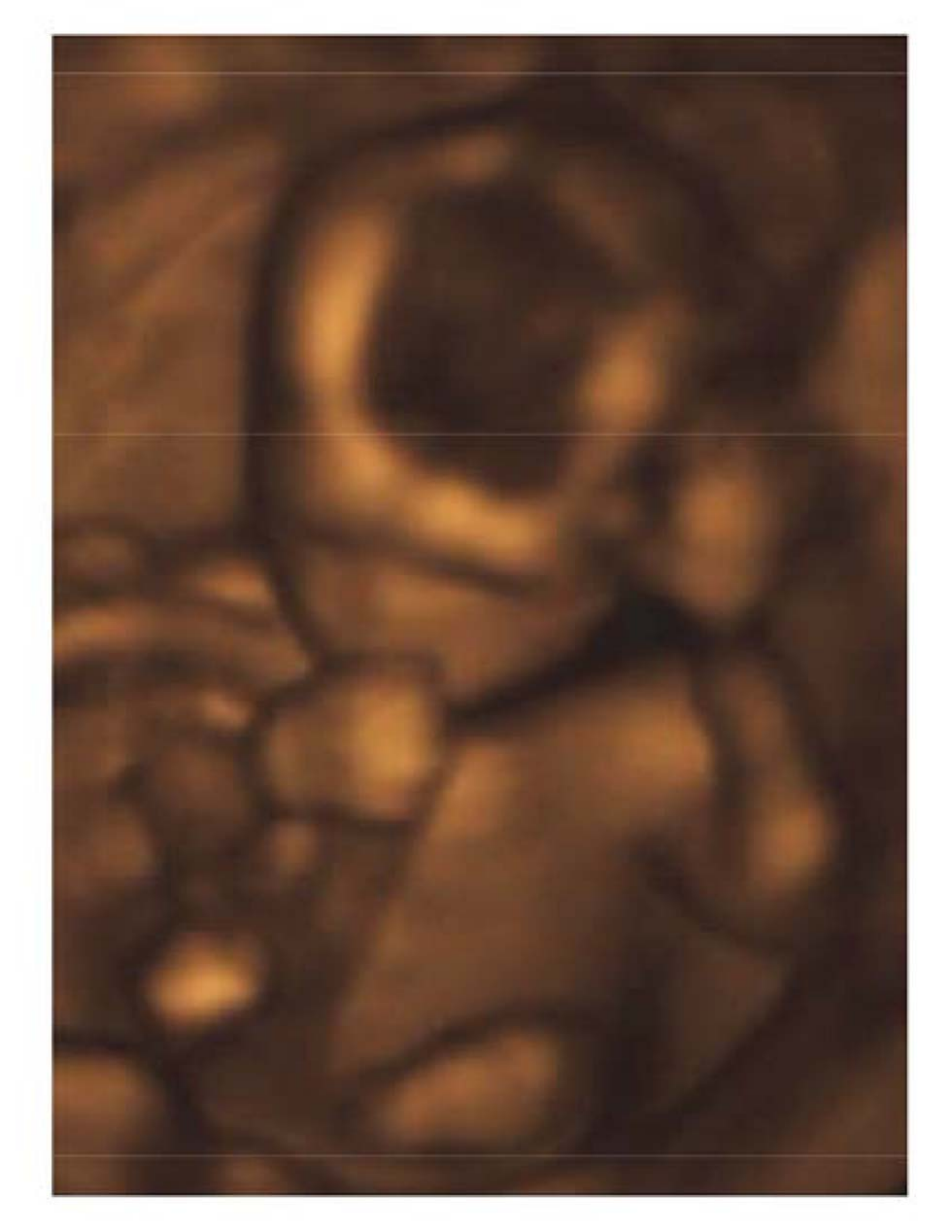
Ultrasound image of a fetus sucking the right thumb. Image credit: jenny cu (http://commons.wikimedia.org/wiki/File:Sucking_his_thumb_and_waving.jpg).
